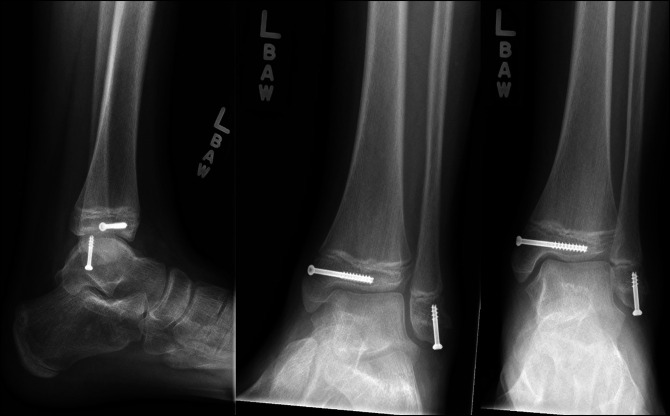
AP, mortise, and lateral view ankle radiographs 2 months after surgical fixation demonstrating complete healing of fractures.
